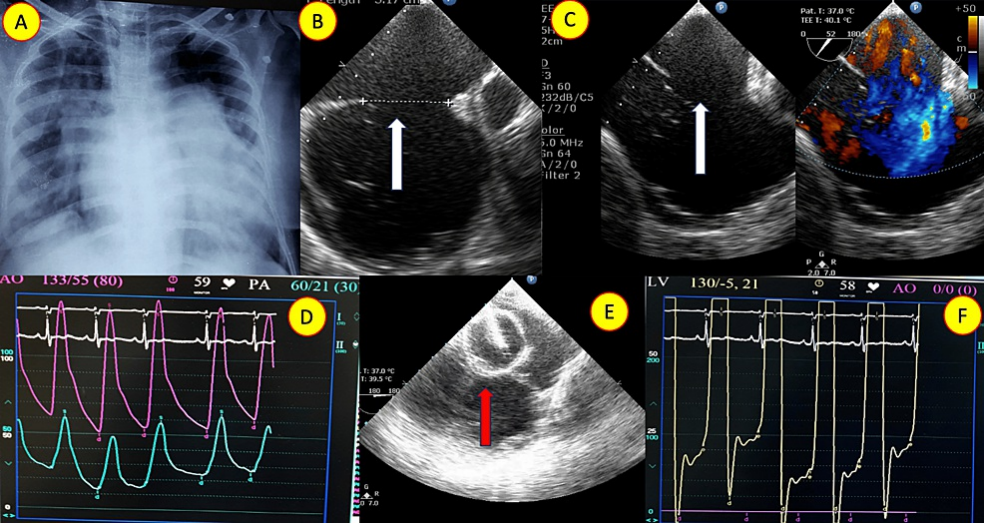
Secundum atrial septal defect with left ventricular diastolic dysfunction and pulmonary hypertension A: Chest X-ray (posterior-anterior view) showcasing significant cardiomegaly and a dilated main pulmonary artery. B, C: Transesophageal echocardiogram with color Doppler revealing a large secundum atrial septal defect (ASD) with bidirectional shunt (white arrow). D: Hemodynamic tracing demonstrating elevated pulmonary artery pressure. E: Balloon occlusion of ASD (red arrow) resulting in a substantial increase in left ventricular end-diastolic pressure (F).
